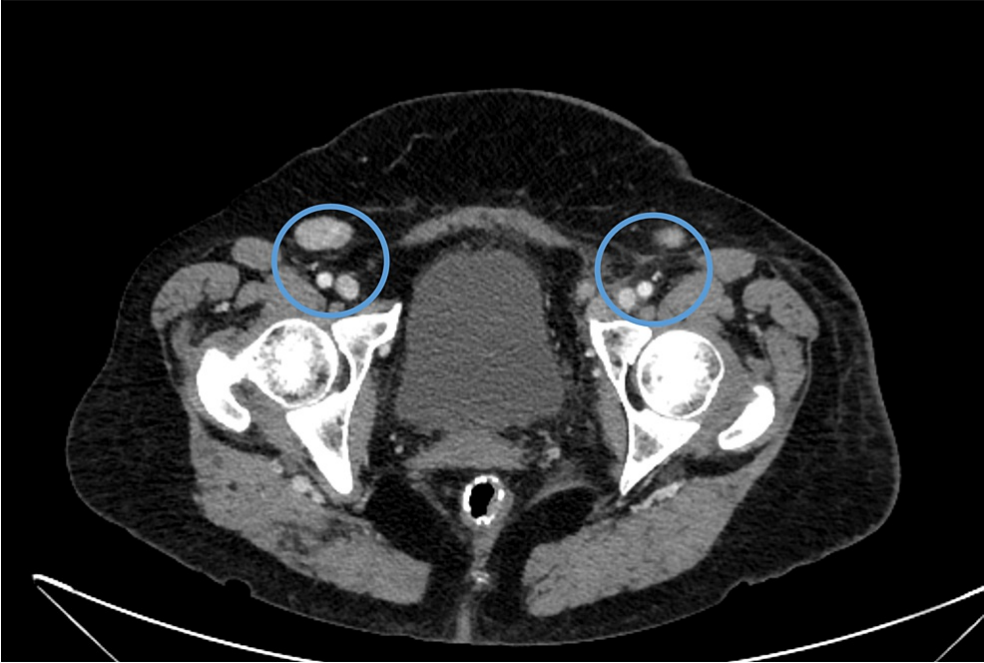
Enlarged lymph nodes in the pelvic CT scan CT: computed tomography
